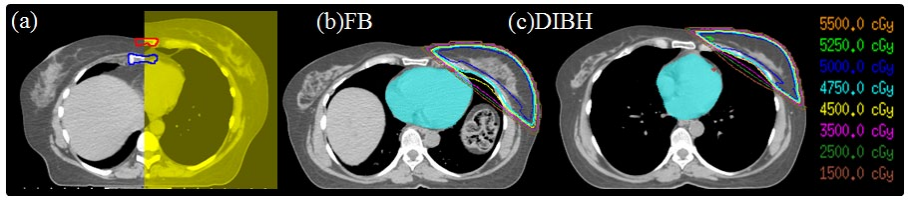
Anatomy‐based registration (a) of the two CT datasets, DIBH and FB. Contours of the sternum are shown: the red contour is on the DIBH dataset (yellow) and the blue one is on the FB dataset (gray). Isodose lines from plans on a sample patient on the (b) FB and (c) DIBH planning studies are shown. Organs at risk (OARs) were contoured (blue colorwash = heart,red colorwash = LAD).
